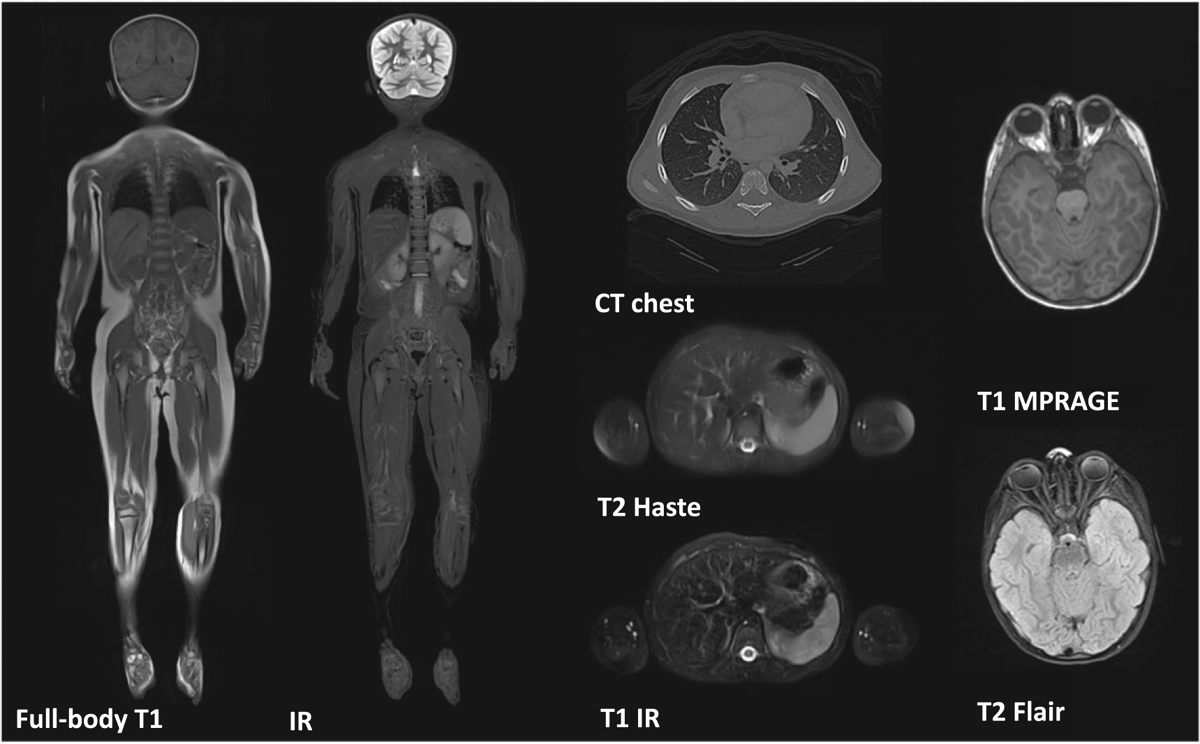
Structural MRI and CT scans used for the segmentation process. Different scans are used to visualize the body tissues with various levels of detail. We used full body T1 and IR images to segment the female model’s tissues from head to toes. In addition, we used T2 HASTE, T1 IR, and CT images of the chest and upper abdomen to extract further information about the anatomical structures of this body compartment. We also used T1 MPRAGE and T2 Flair Images for the brain segmentation.
